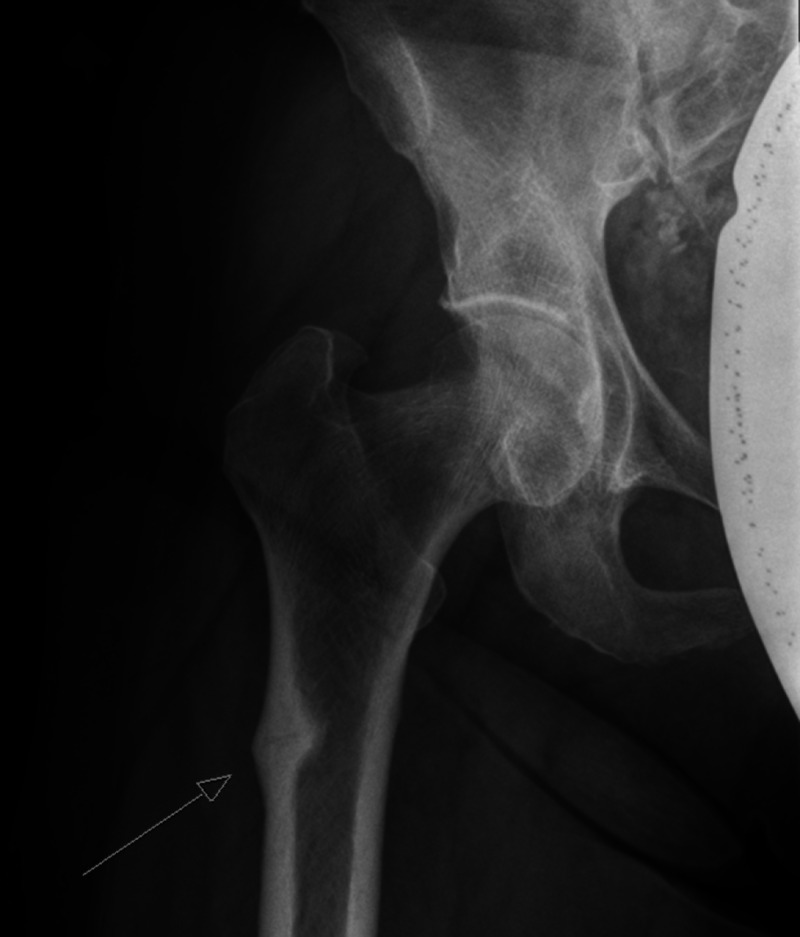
X-ray right hip shows a right atypical femoral fracture The lateral cortical periosteal thickening and oblique fracture is more pronounced.
